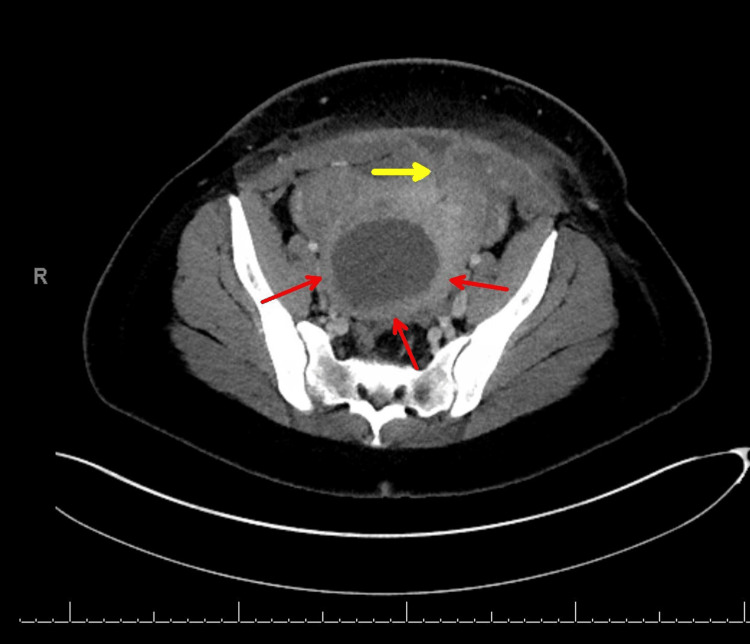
Transverse CT scan on the pelvis (day 65 after initial admission and 50 days after cesarean delivery). There is a persistent fluid collection in the left ventral abdominal wall concerning for an abscess (yellow arrow). Possible fistulous tract between the abdominal wall collection and myometrium, possibly in continuity with the uterine abscess, versus a degenerating fibroid. The uterine wall is indicated by the red arrows.
